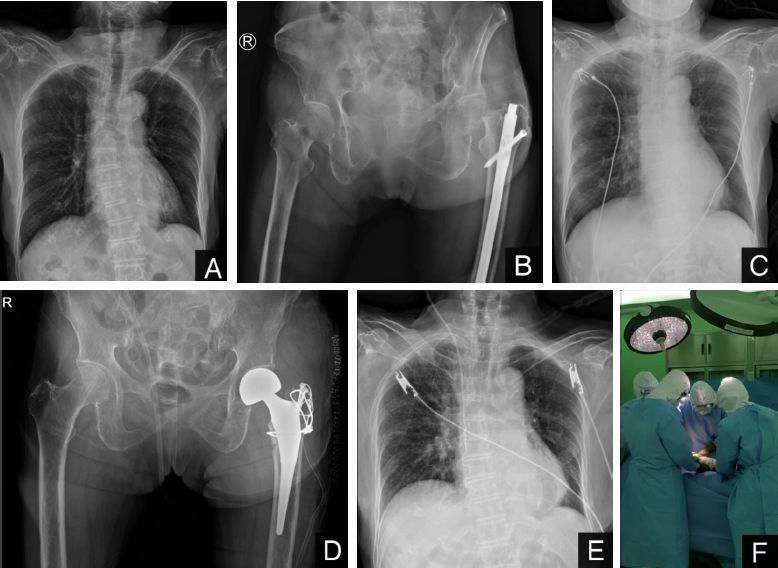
Chest radiographs (Panels A and C) obtained in a local clinic (A) and after presentation to our hospital (C) showing no active pulmonary lesions. Panel B shows a left femur neck fracture. Panels D and E are plain radiographs taken the day after surgery. Panel F shows the surgical team performing surgery while wearing level D PPE.
